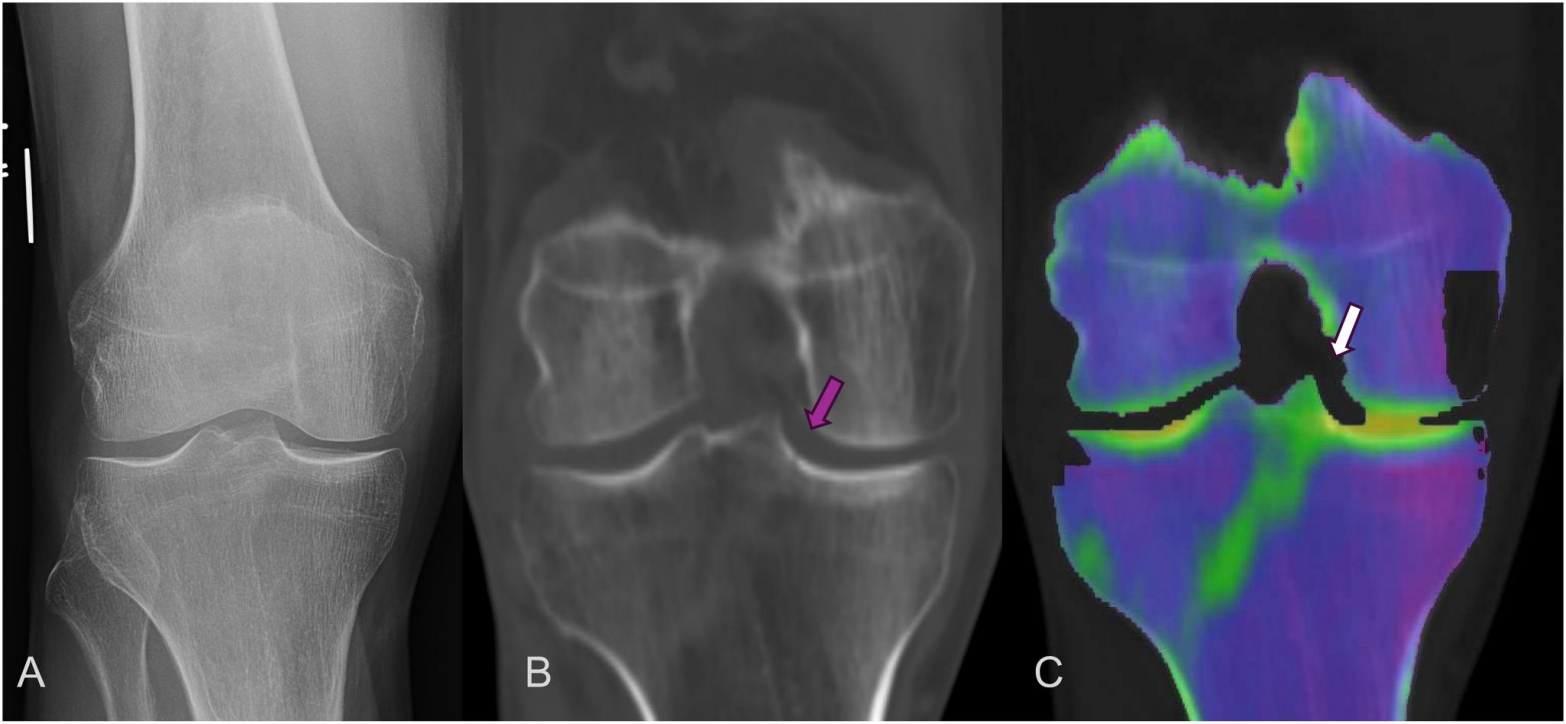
The radiograph of the patient presented in ER was negative for any acute fracture (A) however DECT was performed which showed an incomplete fracture on CT (B) but on dual energy map there was linear marrow edema involving the lateral tibial plateau (C).
